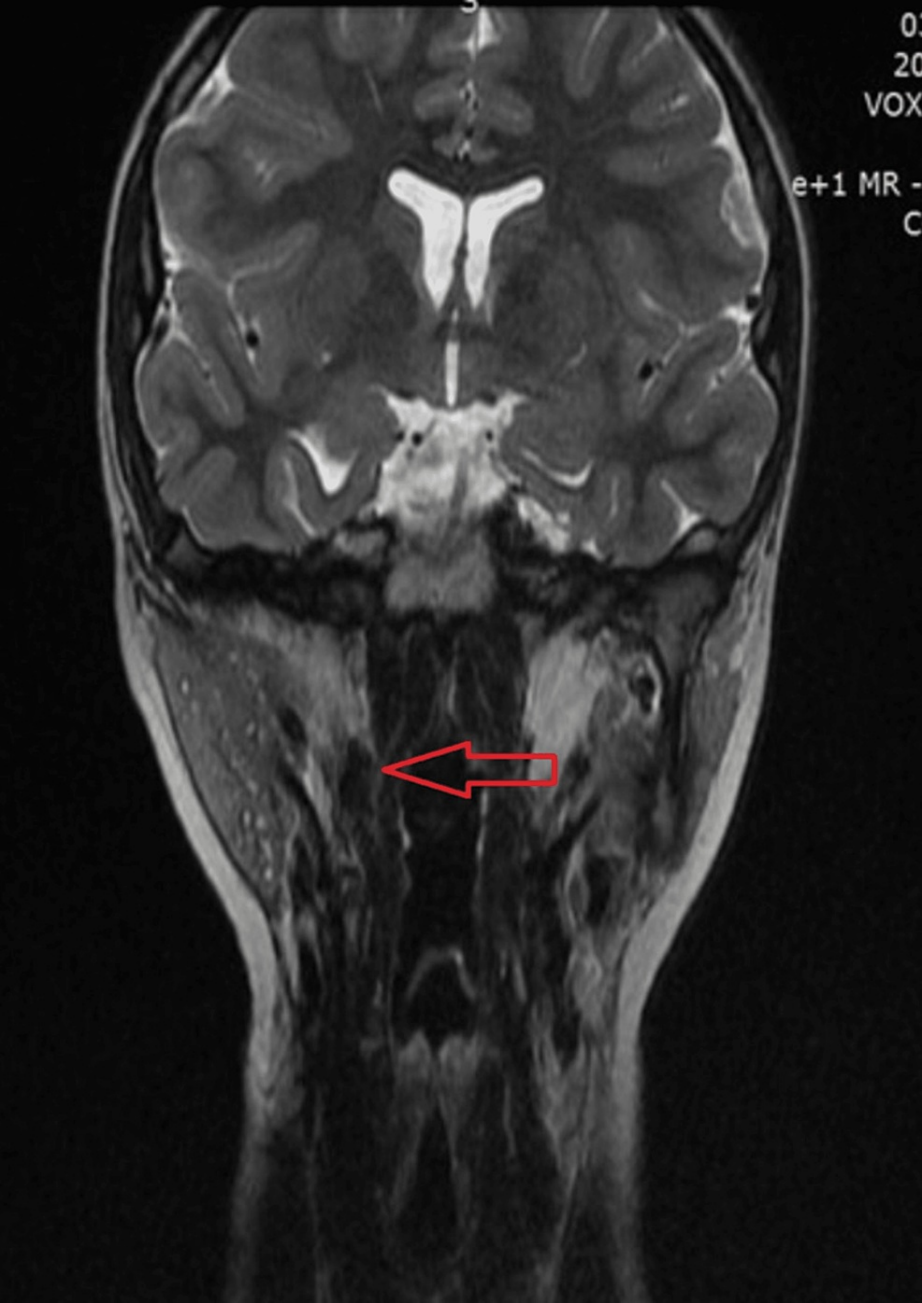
T2-weighted images one year after ectopic thymus resection showing complete removal of the lesion. Red arrow shows the post-operative area.
